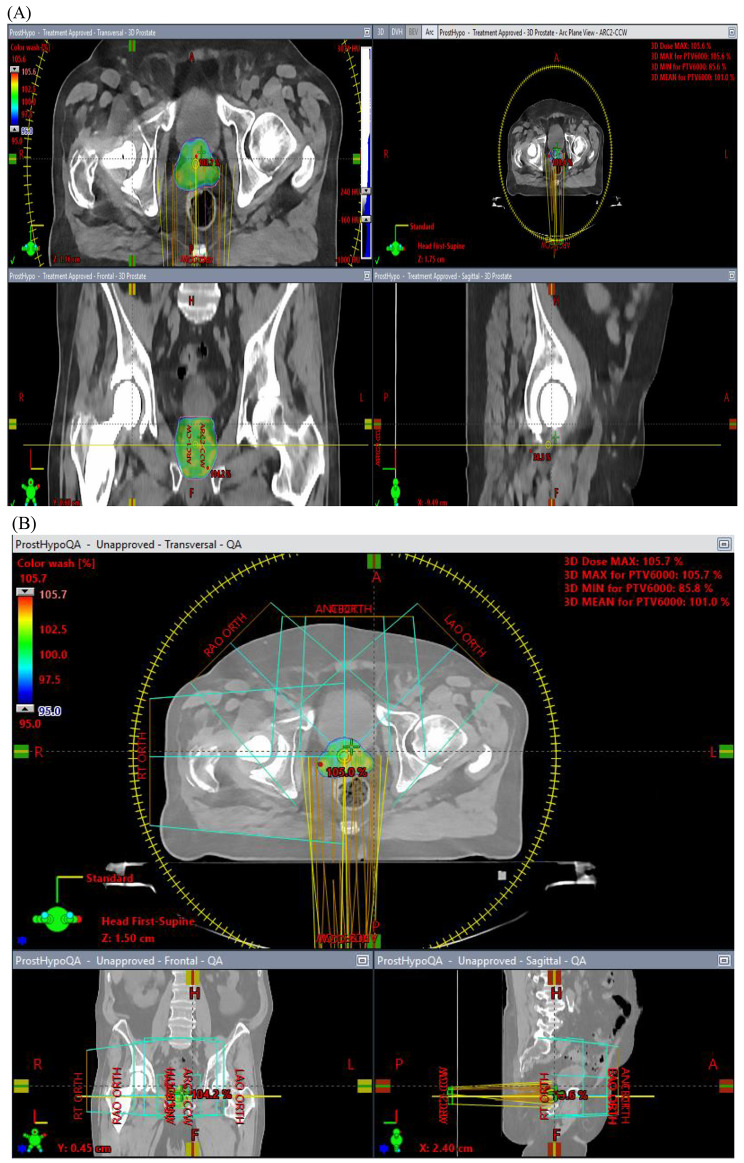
Axial contrast-enhanced CT scans of a 76-year-old man with a right metal hip prosthesis who underwent radiation treatment for intermediate-risk prostate cancer (A) and the imaging on quality assurance (B)
